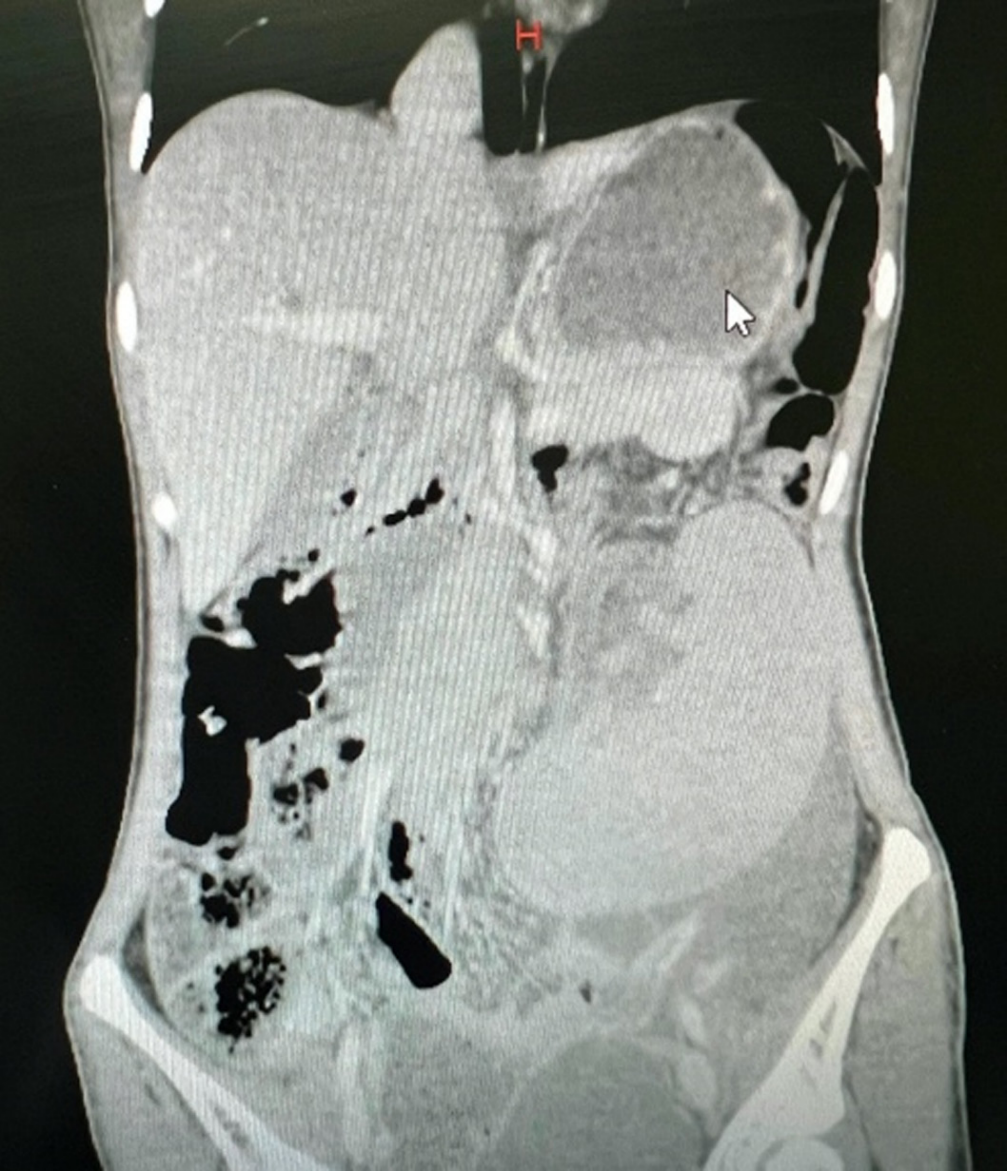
Computed tomography of patient, revealing a mispositioned spleen before surgery.
